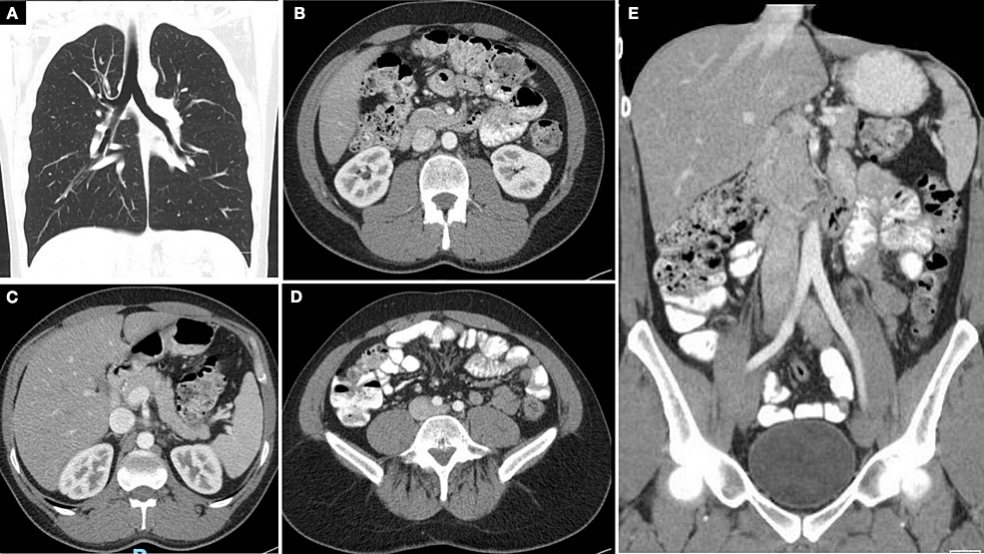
TAP CT scan without evidence of hepatic, pulmonary, and lymph node metastases. (A) Coronal axis view of the lungs. (B), (C), (D) Axial and coronal (E) axis views of the abdomen and pelvis. TAP CT: computer tomography of the thorax, abdomen, and pelvis
